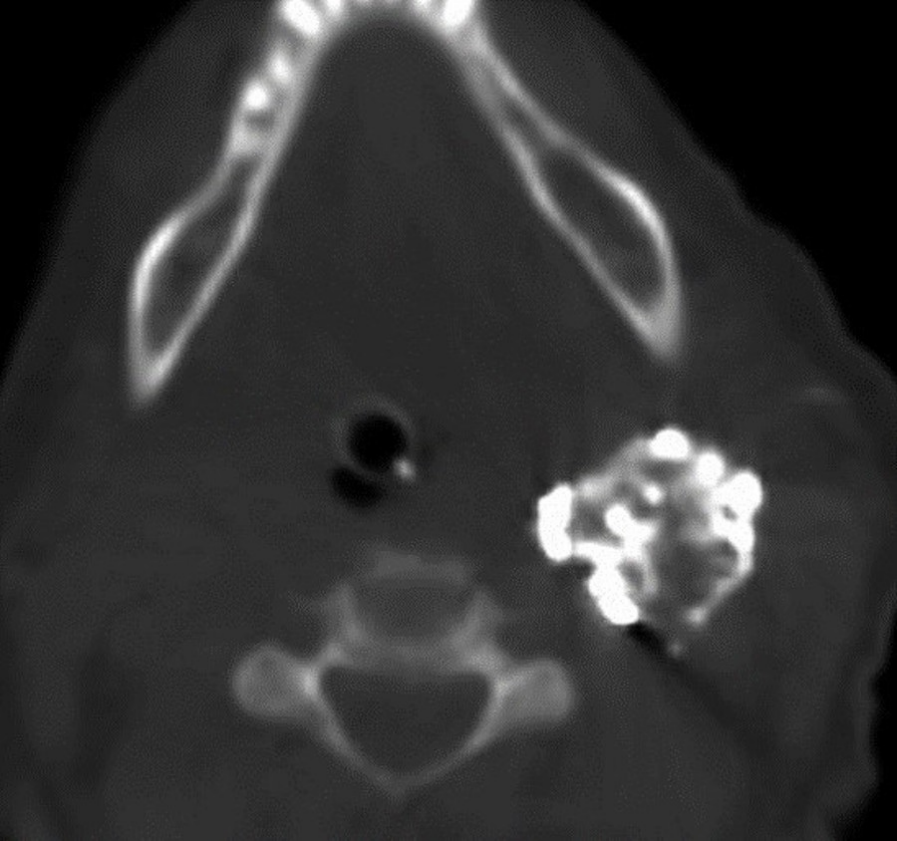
Post-treatment axial XPER CT detailing the Onyx mass within the tumor.
